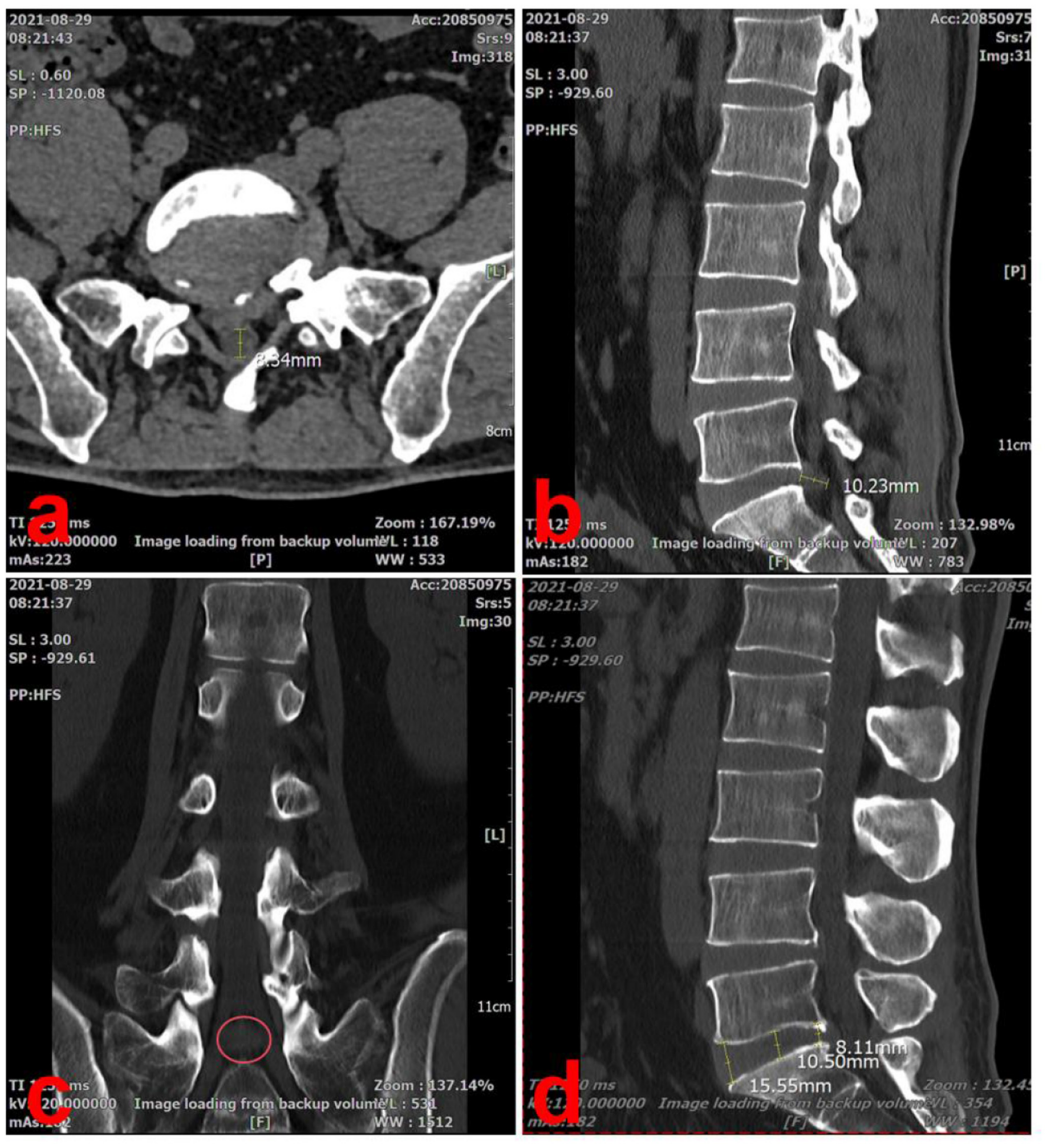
Lumbar spine CT scan at the initial visit (2021-08-29). (a) On the cross-section view, the diameter of the spinal canal at the L5/S1 level was 8.34 mm. (b) On the sagittal view of the L5/S1 intervertebral space, the herniated disc measured 10.23 mm in length. (c) The coronal view, the herniated disc was compressing the right S1 nerve root, consistent with the patient's symptoms (red circle). (d) On the sagittal view, the height of the lumbar intervertebral space was 11.83 mm.
